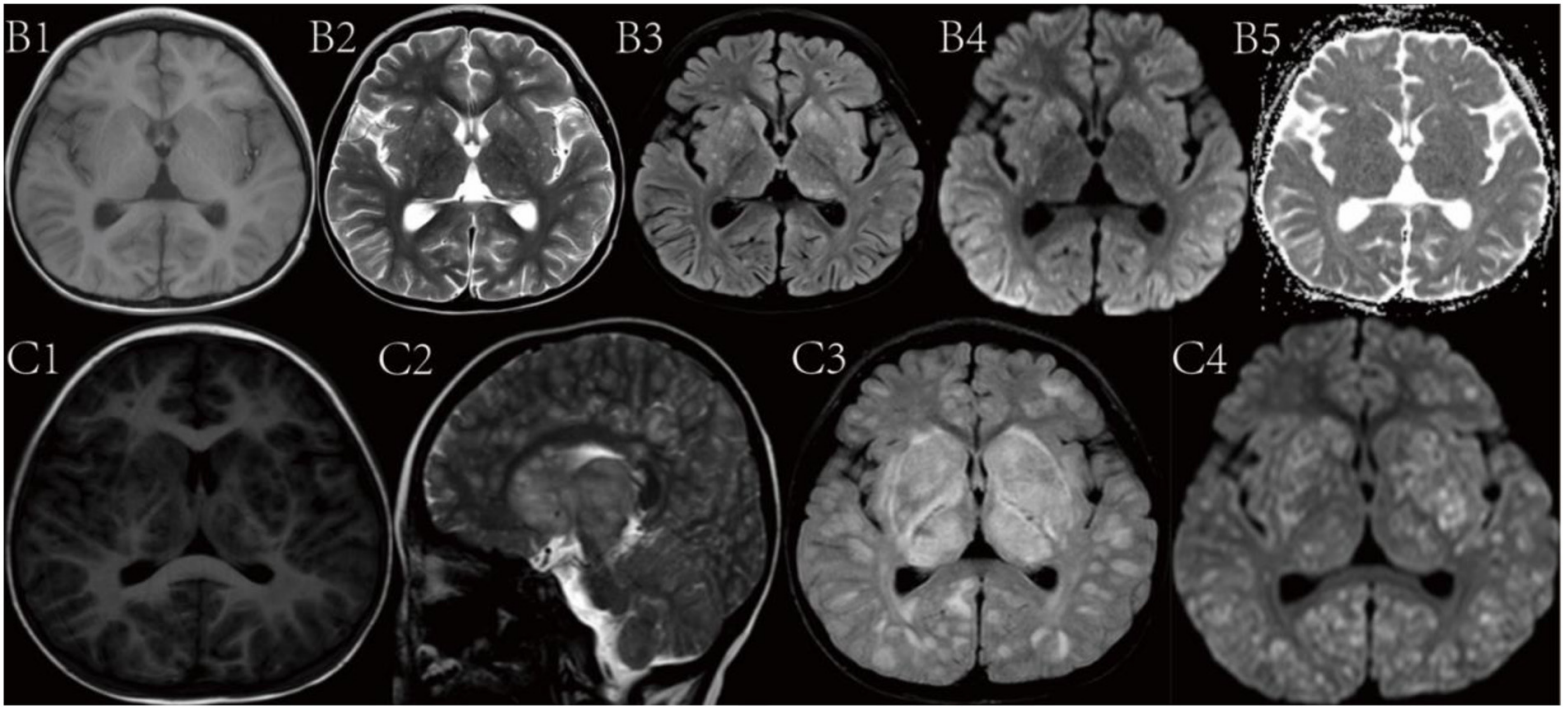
Brain magnetic resonance imaging findings for patient 2 upon initial presentation at +54 days of transplant (B1-B5) and 6 days later at +60 days of transplant (C1-C4). Images from B1 to B5 showing multiple punctate and small nodular lesions less than 0.5 cm in size in bilateral cerebral hemispheres, basal ganglia, and thalamus, with indistinct margins upon presentation. Images from C1 to C4 showing worsening lesions with significant increase in size and number of the brain lesions. Brain magnetic resonance imaging including the T1-weighted (B1, C1), T2-weighted (B2, C2), T2-fluid attenuation inversion recovery (FLAIR, B3, C3), diffusion-weighted imaging (DWI, B4, C4), and apparent diffusion coefficient images (ADC, B5).
